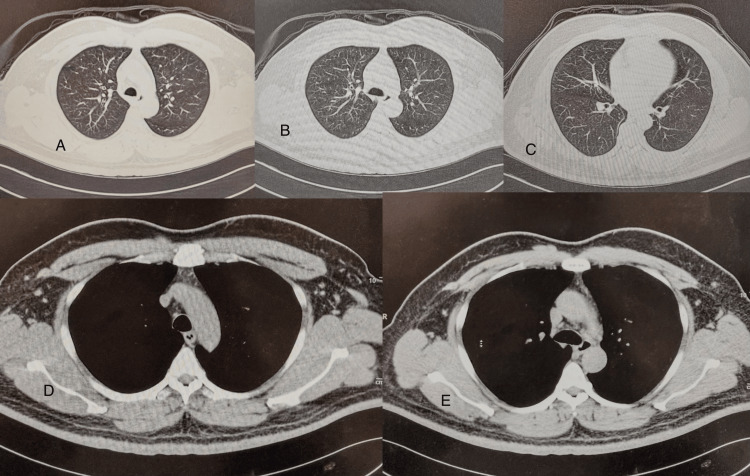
Contrast-enhanced CT thorax after treatment (A, B, and C) Follow-up CT images in the lung window demonstrate complete resolution of the previous lesions. (D and E) Follow-up axial CT images in the mediastinal window show complete resolution or significant regression of previously noted mediastinal lymphadenopathy, with the paratracheal (D) and precarinal lymph nodes (E) returning to normal size. CT: computed tomography
